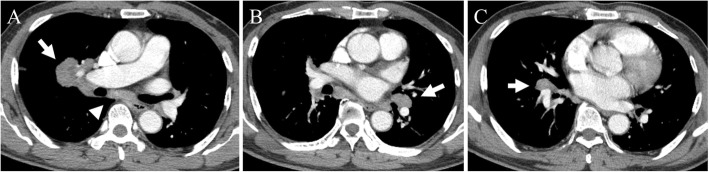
Chest CT scans at 5 months after diagnosis of sarcoidosis. a Aggravating right hilar lymphadenopathy (arrow) and no interval change of subcarinal lymphadenopathy (arrow head). b No interval changes of left interlobar lymphadenopathy (arrow). c No interval changes of right interlobar lymphadenopathy (arrow)
